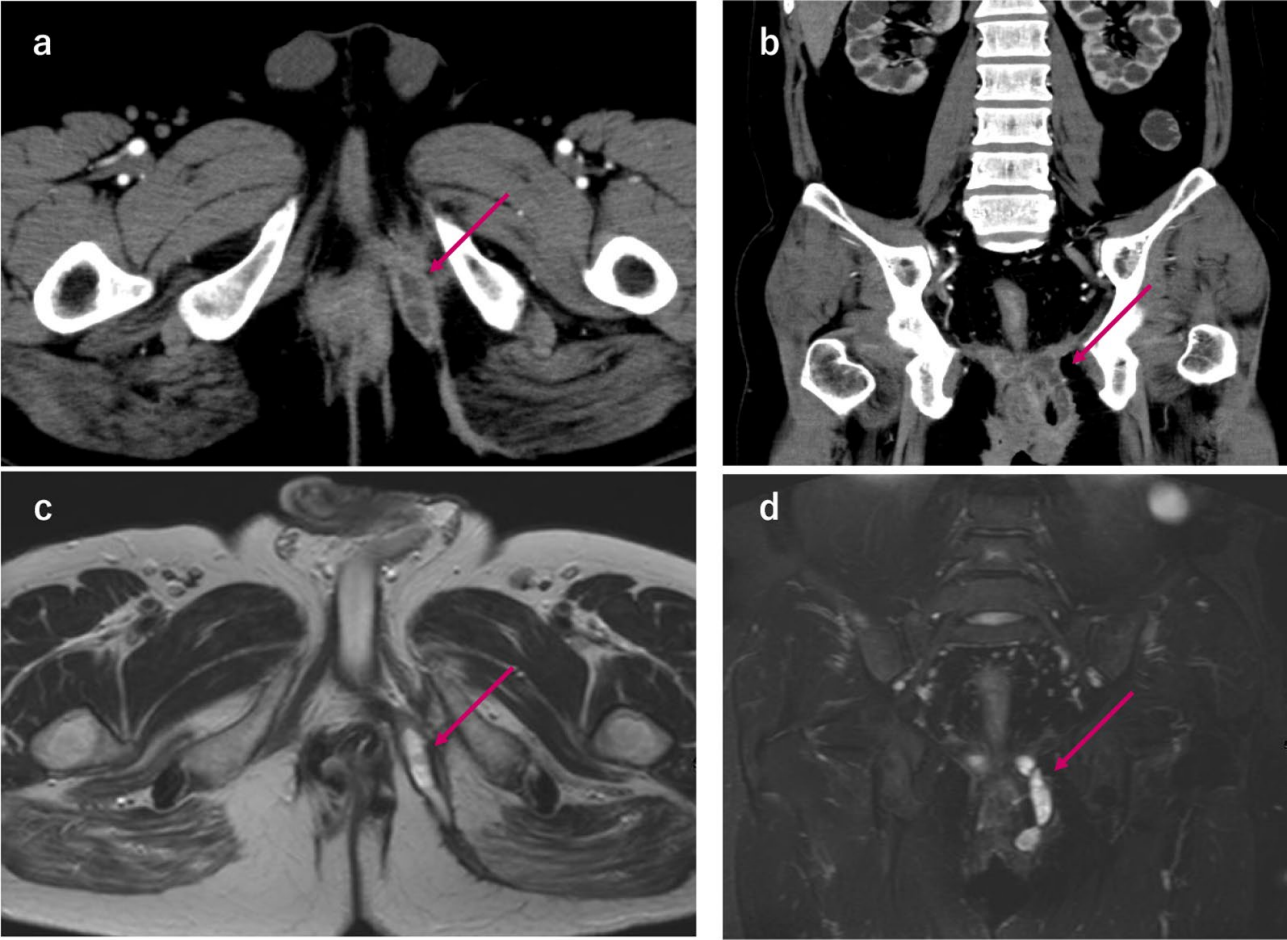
Abdominal CT (a, b) and MRI (c, d) show the presence of anal fistula (arrow) to the buttock subcutaneous from the front of sacrum
